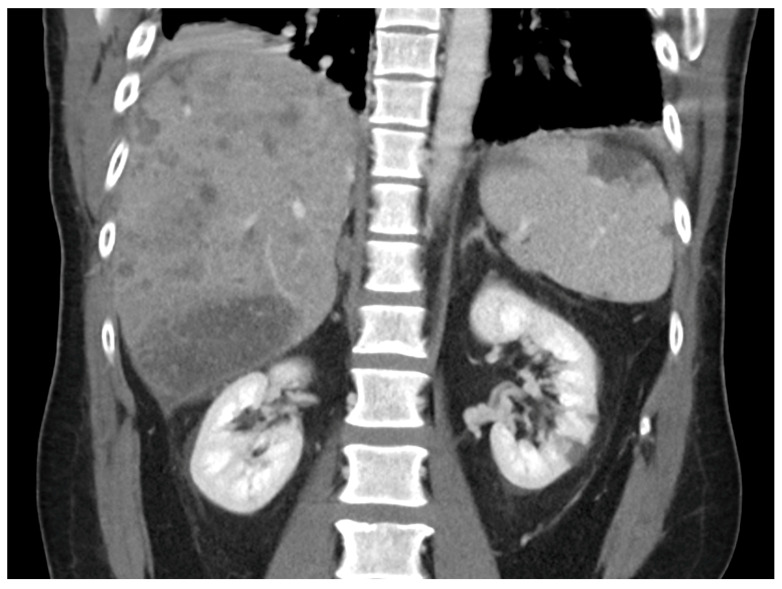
Abdominal CT: Abdominal CT with IV contrast showing acute splenic and bilateral renal infarcts suggestive of an embolic phenomenon. Diffuse hepatic metastasis can also be observed.
